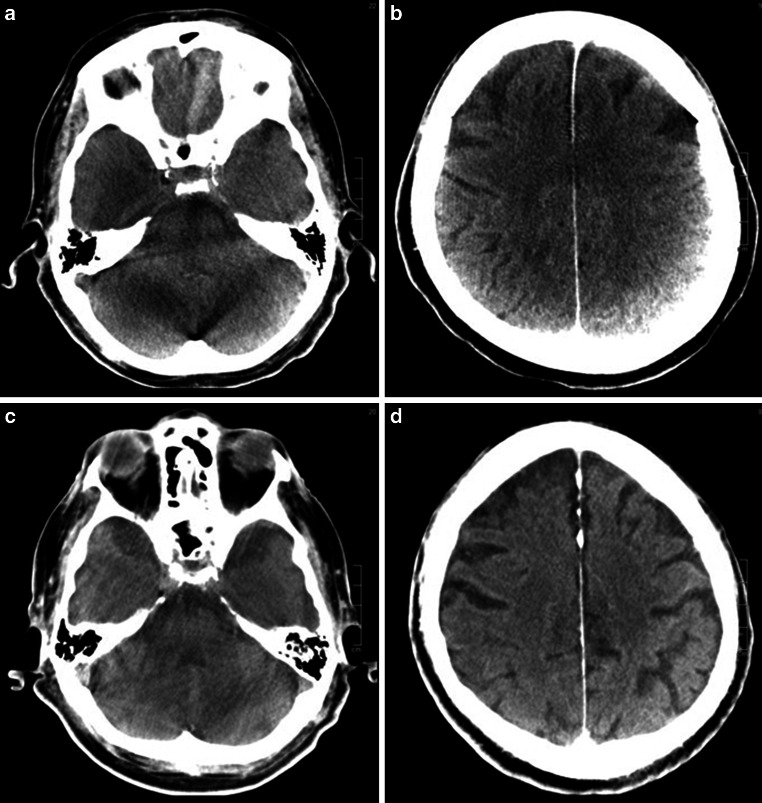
Upper row* (a/b) shows infratentorial and frontoparietal slices taken from circular FP-CT. Note marked artifacts from the skull base, petrous bones and internal occipital protuberance (a) and inhomogeneous contrast especially in the parietal lobes (b). *Lower row* is taken from sinusoidal FP-CT of a different person. Note reduced posterior fossa artifacts with better visualization of brainstem and 4th ventricle (c) and more homogeneous contrast of the cerebrum with improved differentiation of grey and white matter (d)
